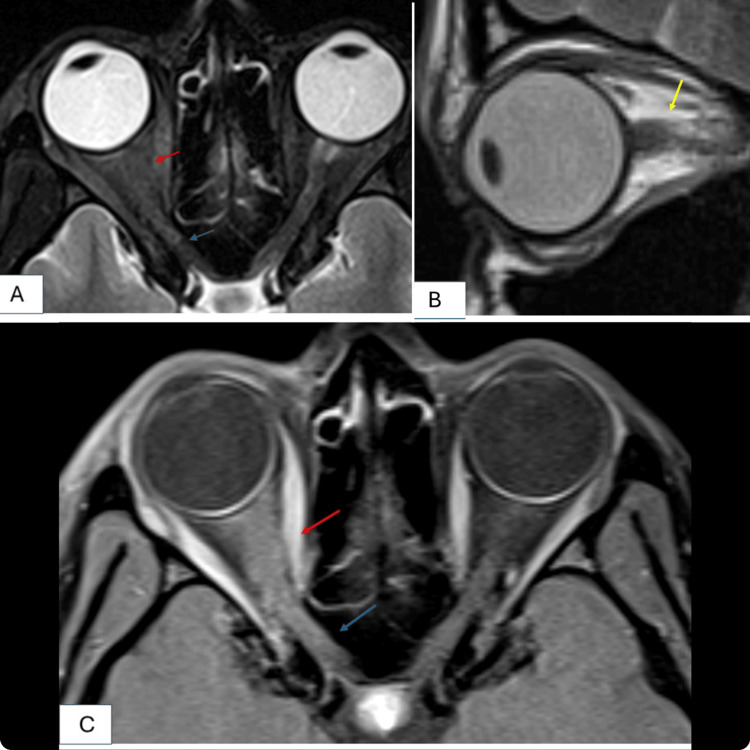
Neuroimaging A) Axial T2-weighted image of the MRI brain and orbit reveals a thickened intraconal (red arrow) and intracanalicular (blue arrow) part of the right optic nerve sheath complex. B) Sagittal T2-weighted image showing hyperintensities posterior to the globe, with surrounding fat stranding (yellow arrow). C) Axial T1-weighted post-contrast image showing homogeneous enhancement of the thickened intraconal (red arrow) and intracanalicular (blue arrow) part of the right optic nerve.
